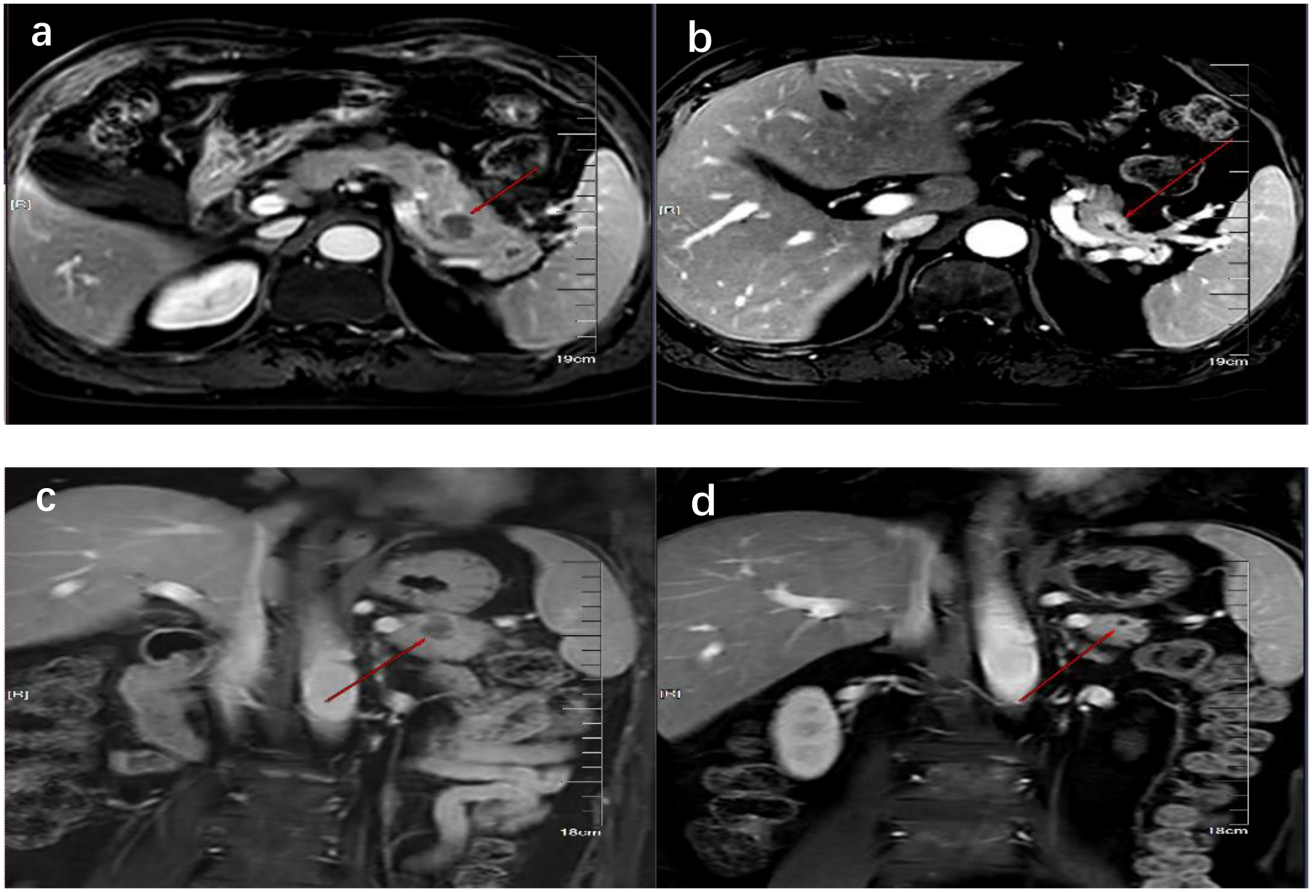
MRI showed the comparison of pancreatic lesions before (A, C) and after (B, D) the treatment of chemotherapy. (A, B) are MRI images of horizontal pancreas before and after treatment, and (C, D) are MRI images of coronal pancreas before and after treatment. The red line arrows point to the location of pancreatic lesions before and after treatment.
